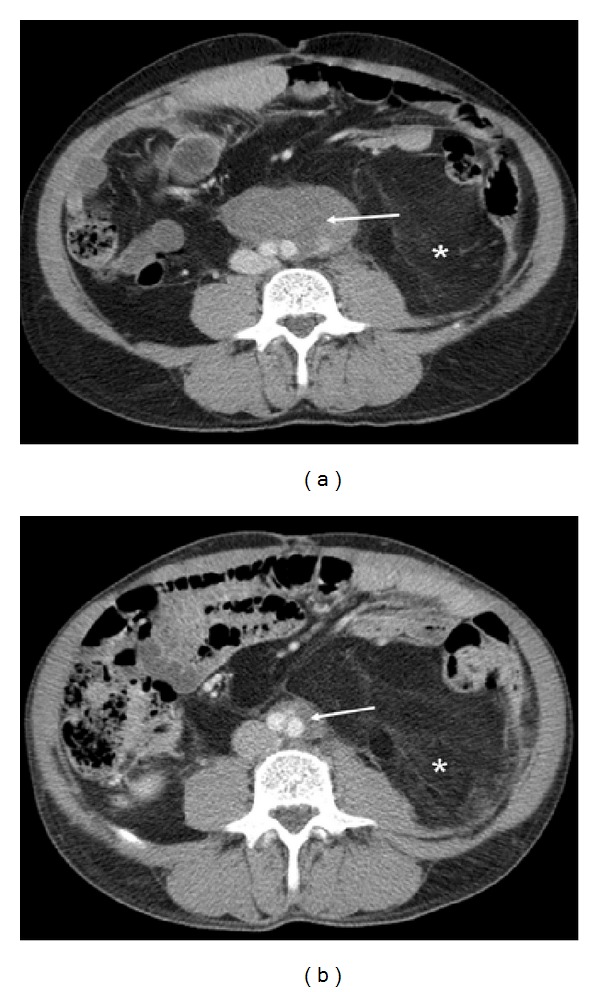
Axial contrast enhanced CT images of the abdomen in a 63-year-old male with retroperitoneal liposarcoma at baseline (a) and following 5 months therapy (b). Although the well-differentiated component filling the left side of the retroperitoneum (∗) showed little change, the dedifferentiated component (arrows) surrounding the aortic bifurcation reduced in size from 7.9 cm to 2.5 cm (PR).
